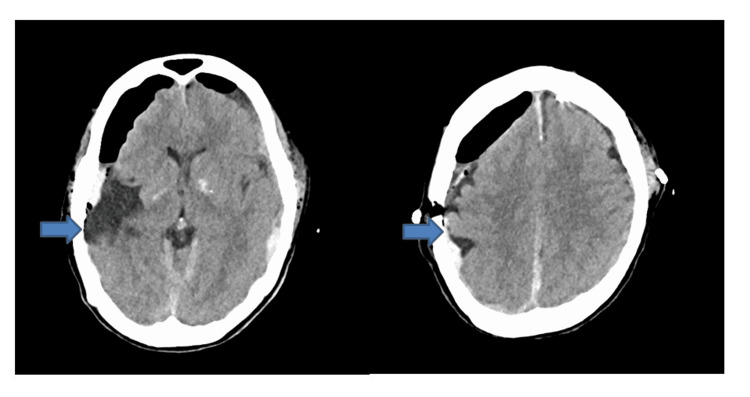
Postoperative CT obtained 12 hours after arrival (after the second surgery) confirmed complete removal of the right hematoma (arrow) and resolution of the midline shift.
